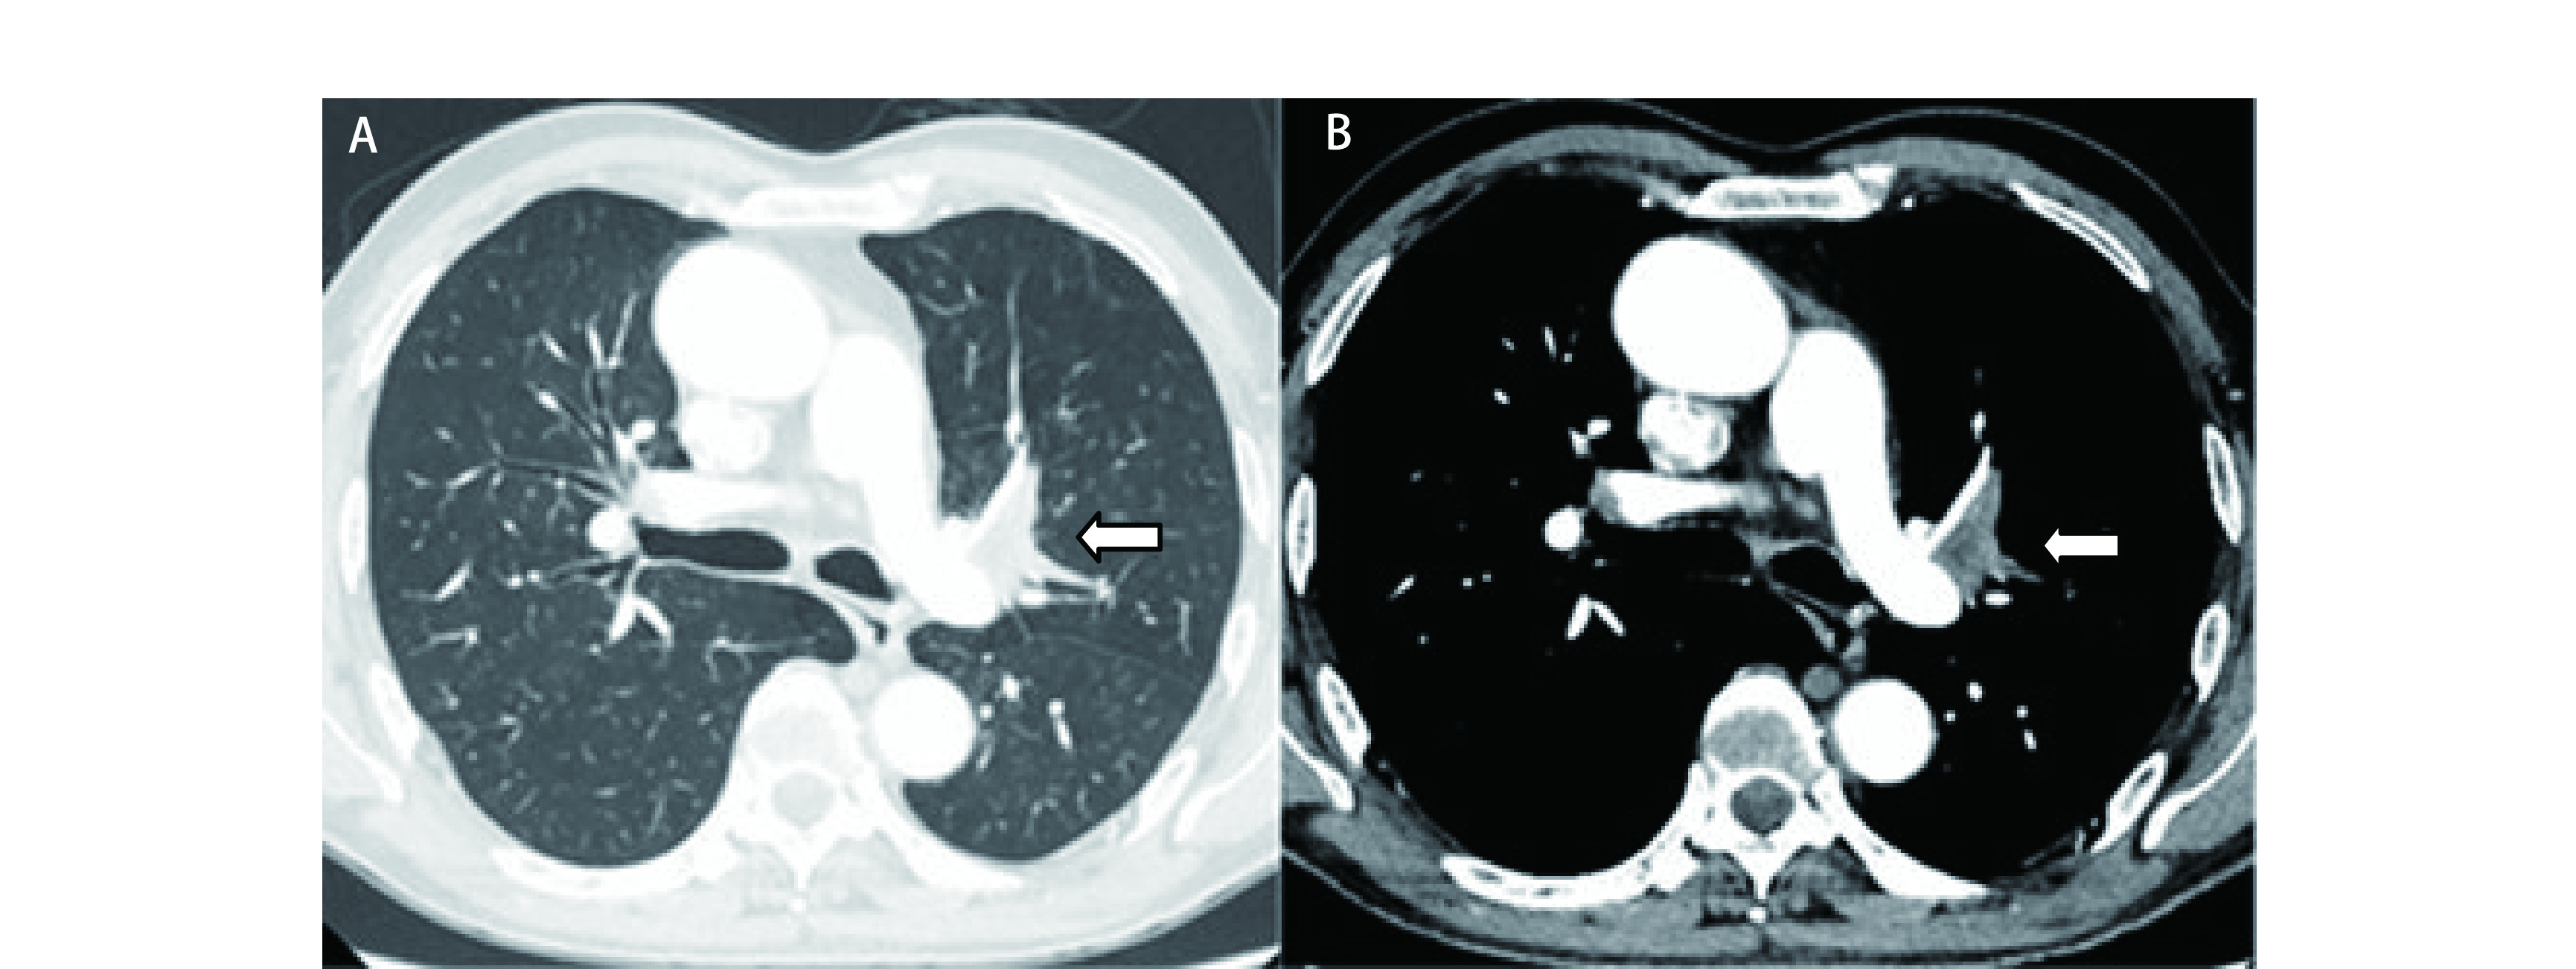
胸部增强CT检查。A：肺窗, 左肺上叶中央型肺癌(白色箭头所示); B：纵隔窗, 肿瘤侵犯肺动脉干, 肿瘤35 mm×21 mm×15 mm(白色箭头所示)。 Thoracic enhanced CT scanning. A: pulmonary window: The tumor is located in the center of the left upper lobe (as indicated by the white arrow); B: Mediastinal window: The tumor invades the the left pulmonary artery, the tumor size is 35 mm×21 mm×15 mm (as indicated by the white arrow).
